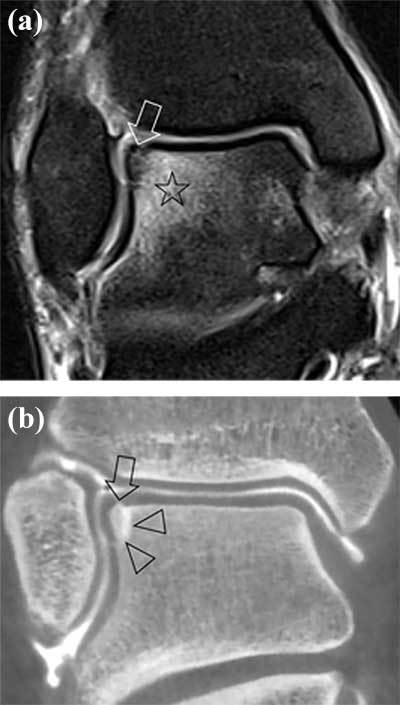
Stage 3 according to Anderson classification. Coronal PD fat suppressed coronal image (a) showing an OCL fragment completely detached from talar dome without displacement (arrow), bone marrow oedema (star) of adjacent part of the talus. Coronal reformatted CBCT-A (b) demonstrates more clearly the OCL fragment (arrow) separated from the adjacent talus by thin rim of surrounding contrast (arrowheads).
